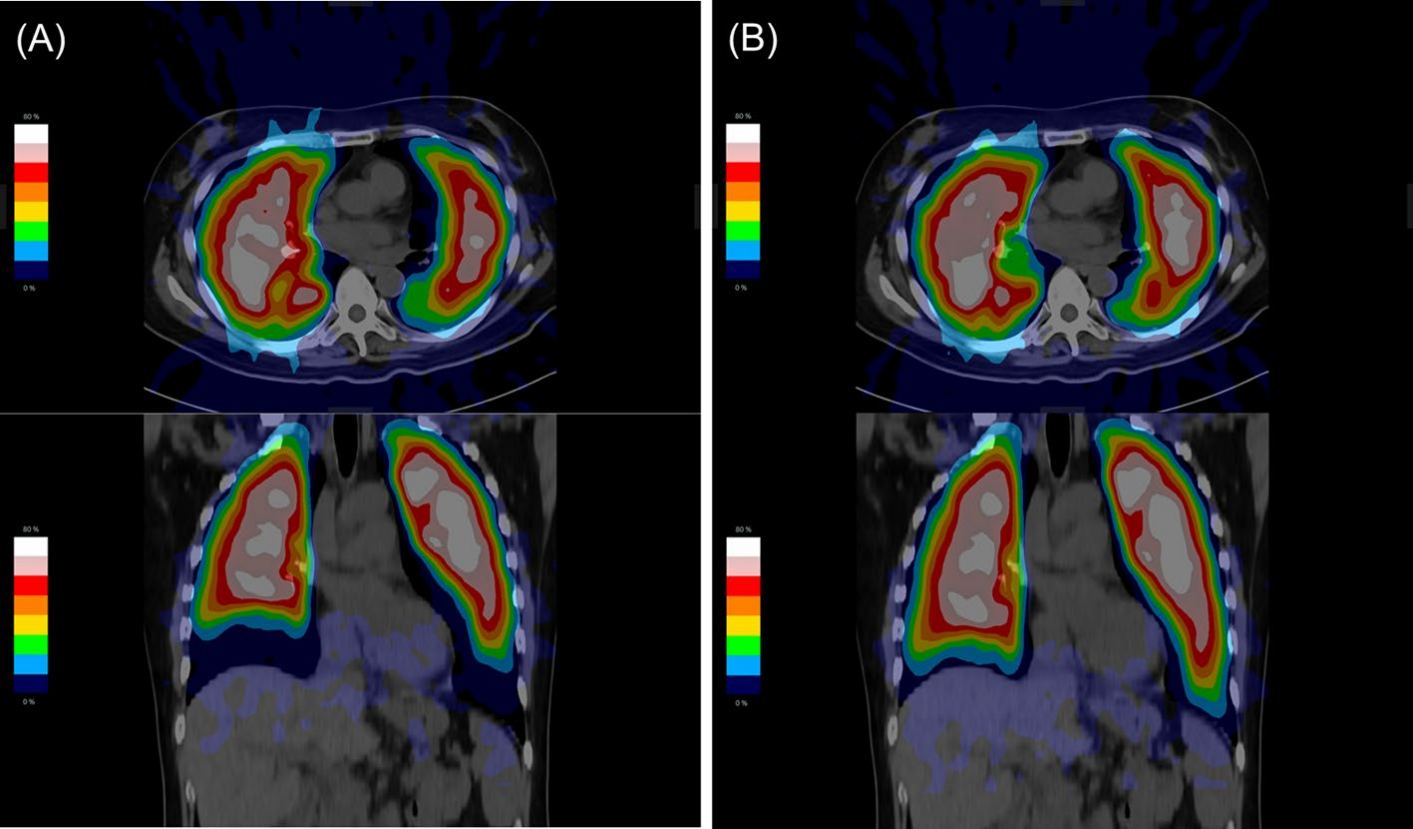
The fusion images between SPECT and CT before and after using the breathing synchronization software. A 50-year-old woman underwent lung perfusion SPECT and CT due to suspected PE; she had no abnormal morphological findings on the CT image and the lung perfusion SPECT image revealed normal lung perfusion. The lung volume calculated using CT was 4332 ml. A Before using the breathing synchronization software, the lung volume calculated using the original lung perfusion SPECT was 3351 ml; B after using the breathing synchronization software, the lung volume calculated using lung perfusion SPECT with the breathing synchronization software was 3974 ml; the volume of lungs calculated from SPECT with the breathing synchronization software was increased, and the conformity between lung perfusion SPECT and lung CT was improved. Abbreviations: CT = computed tomography; PE = pulmonary embolism; and SPECT = single-photon emission computed tomography
